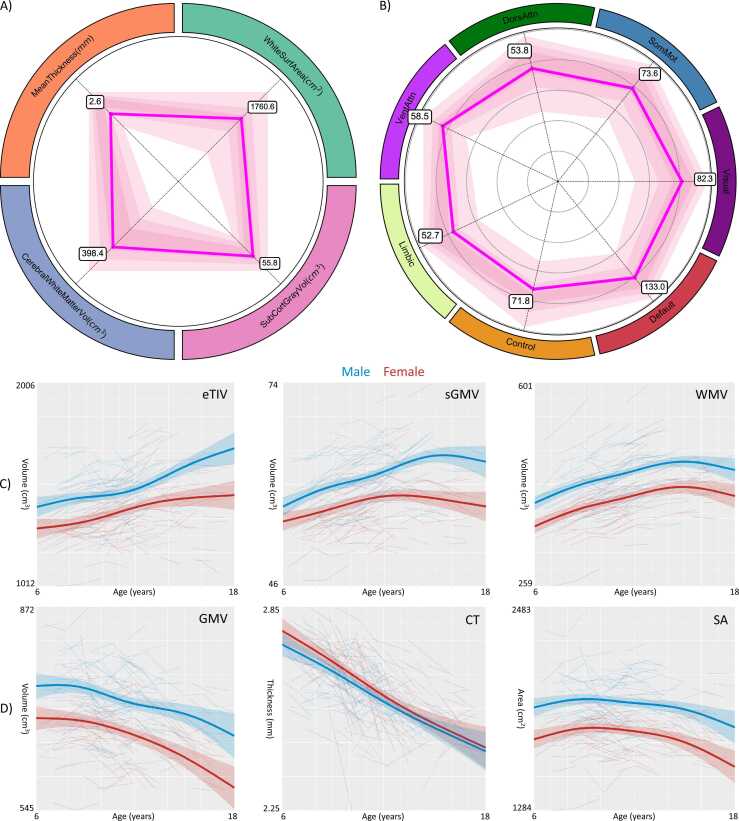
Brain morphological growth curves in schoolchildren. Individual scores are plotted onto the normative growth charts of the global tissue morphological measurements (A) and volumetric measures of the seven common brain networks (see Yeo et al., 2011 for more details on the networks) (B). Growth curves are generated with their confidence intervals for the estimated intracranial volume (eTIV), subcortical gray matter volume (sGMV), cerebral white matter volume (WMV) (C), and total gray matter volume (GMV), mean cortical thickness (CT) and white matter surface area (SA) (D) males and females, respectively. These curves base the growth charts for the individual assessments.
